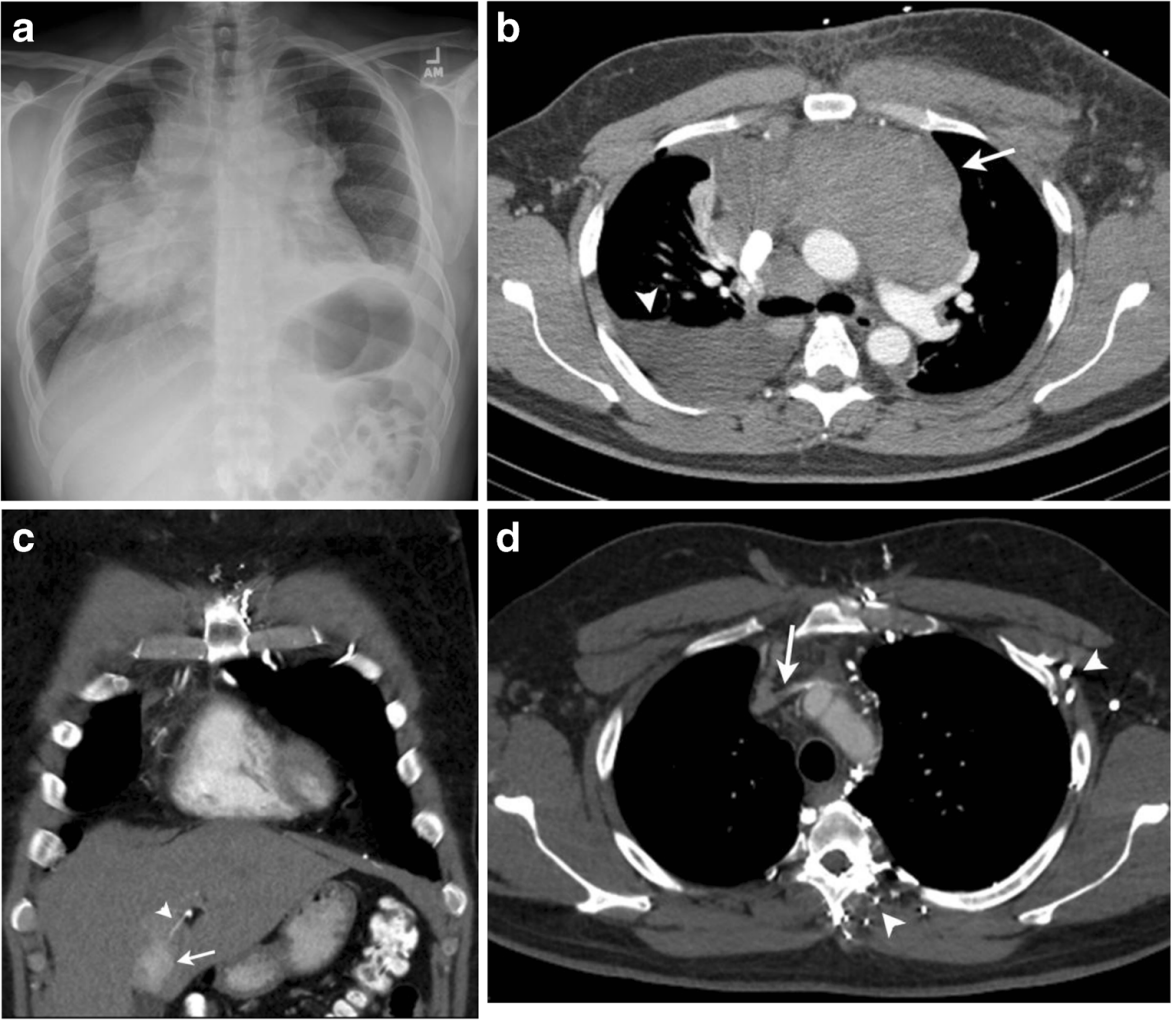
A 39-year-old male with increasing cough, night sweats and weight loss since 2 months. a Frontal chest radiograph demonstrates bulky mediastinal lymphadenopathy exceeding one third of the internal transverse diameter of the thorax. b Axial contrast-enhanced CT image reveals large conglomerate anterior mediastinal lymph nodal mass (*arrow*) and right-sided pleural effusion (*arrowhead*). Biopsy of lymph nodal mass was suggestive of nodular sclerosing HL. Follow-up CT study after treatment with chemotherapy and mediastinal radiation complicated by chronic venous thrombosis. c Coronal contrast-enhanced CT image shows focal hyperenhancement (*arrow*) in segment IV of the liver due to reflux in a capsular vein (*arrowhead*) communicating with the inferior phrenic vein (not shown). d Axial contrast-enhanced CT image demonstrates chronic occlusion of the left brachiocephalic vein (*arrow*) with multiple chest wall and paravertebral venous collaterals on the left side (*arrowheads*)
